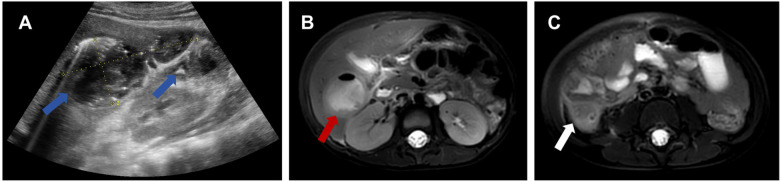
(A) Abdominal ultrasound demonstrates the heterogeneous gas-containing lesion in the right hepatic lobe (blue arrows), indicative of abscess formation. (B) Axial MRI obtained using an early tumor differentiation enhancement protocol shows a low T1, high T2 signal mass in the posterior right hepatic lobe (red arrow). (C) Axial MRI reveals a mass shadow in the appendix region (white arrow), consistent with inflamed appendix.
